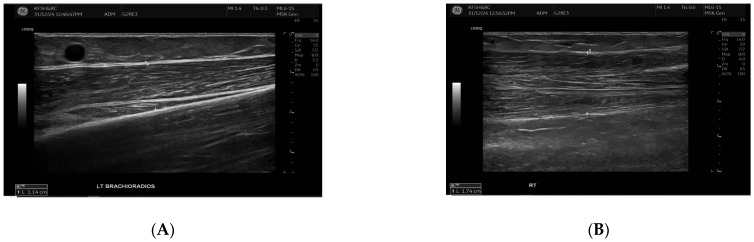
This figure demonstrates the comparison of brachioradialis muscle thickness between the fistula (A) and the contralateral arm (B). B-mode ultrasound images showing brachioradialis muscle thickness measurements. The left panel (A) (fistula side) demonstrates reduced muscle thickness (1.14 cm) compared with the contralateral arm (right panel (B), 1.74 cm). Measurements were obtained in the mid-muscle belly using a linear high-frequency transducer.
